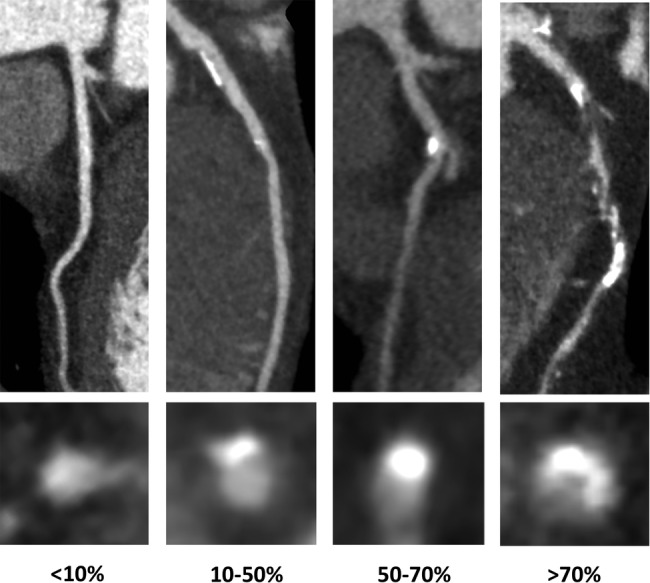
CT coronary angiography curved planar reformations and vessel cross sections showing lesions with different stenosis severity (none, <10%; mild, 10–49%; moderate, 50–70%; severe, >70%).
